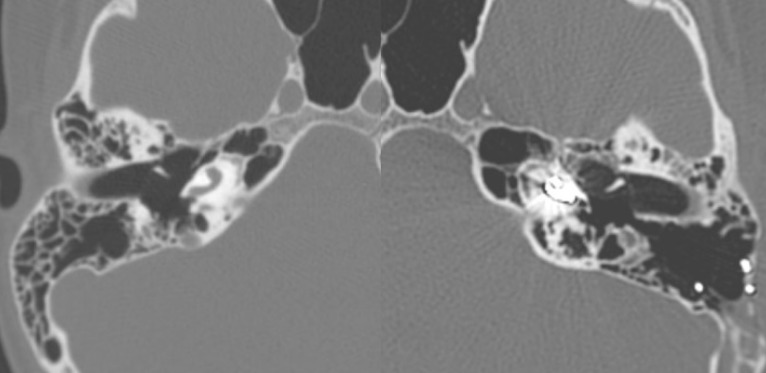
The CT scan of the inner ear in a case having only basal turn of the cochlea (left – preoperatively, right - with folded cochlear implant array inside)
